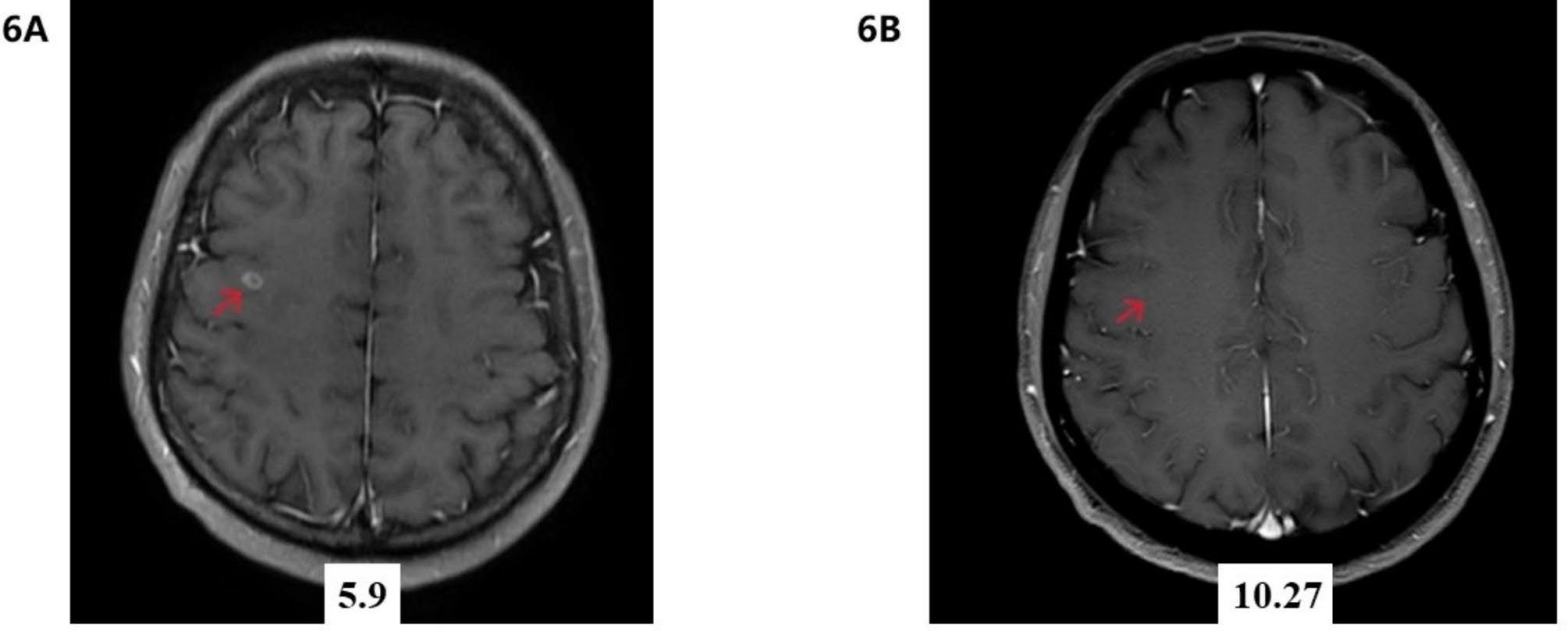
Brain MRI found that abnormal signal in the right frontallobe on May 9th (A), and small metastases has decreased on October 27th (B)
